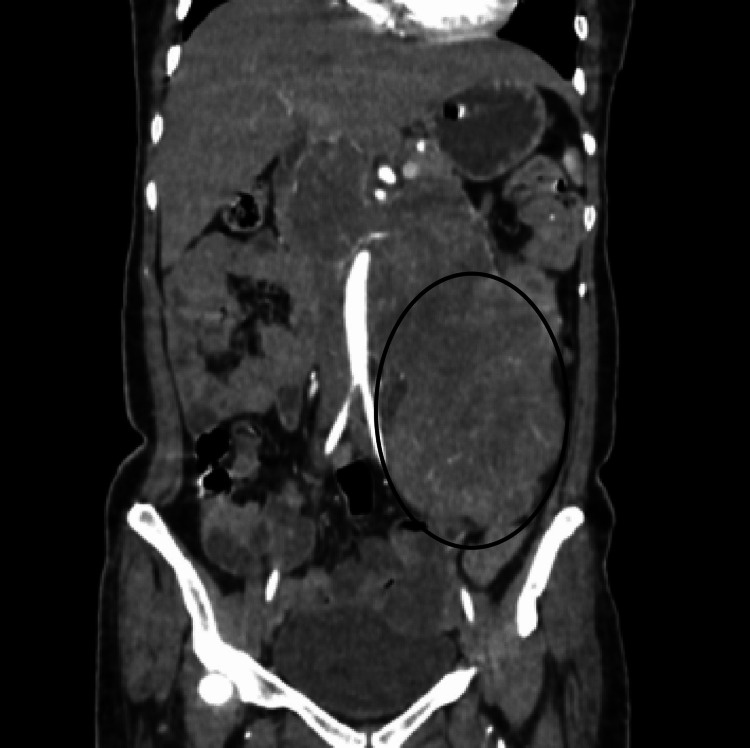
CECT abdomen coronal section showing a well-defined, lobulated, heterogeneously enhancing (circled) mass lesion involving almost the entire left kidney but sparing the upper pole. The lesion shows areas of necrosis. CECT: contrast-enhanced computed tomography
